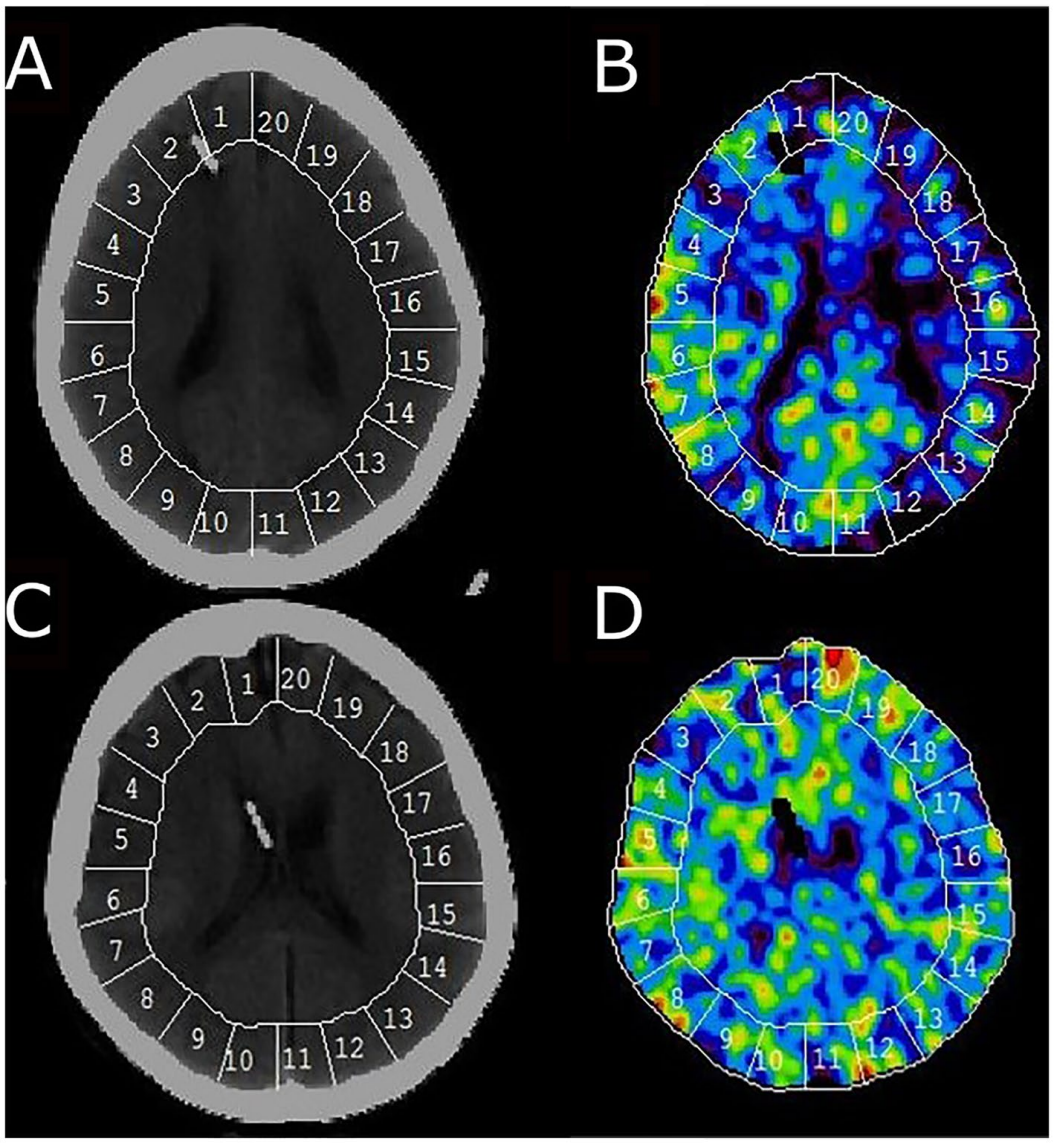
CBF measurements in two patients. The figure demonstrates two Xe-CT scans of two different patients. Patient 1 (a, b) exhibits extensive hypoperfusion particularly in the left hemisphere, whereas patient 2 (c, d) exhibits more normal CBF. CBF, cerebral blood flow, Xe-CT, xenon-enhanced computed tomography
